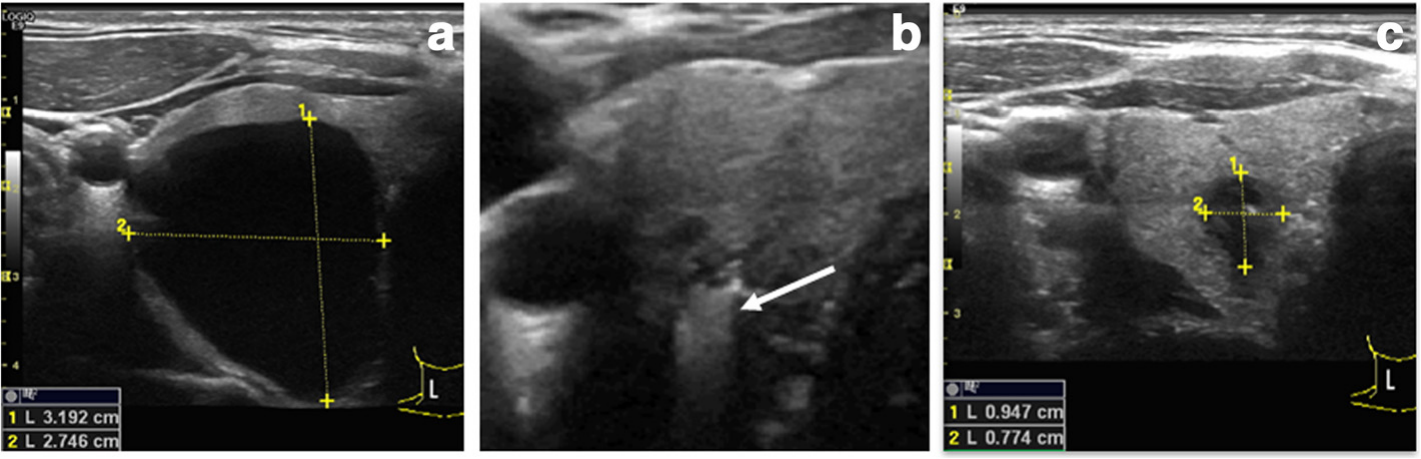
Transverse ultrasound scans of one cyst before (panel a), during (panel b) and after (panel c) percutaneous ethanol injection treatment (PEIT). During PEIT, cystic lumen was filled with instilled ethanol (*arrow*). Panel c shows marked decrease in size
